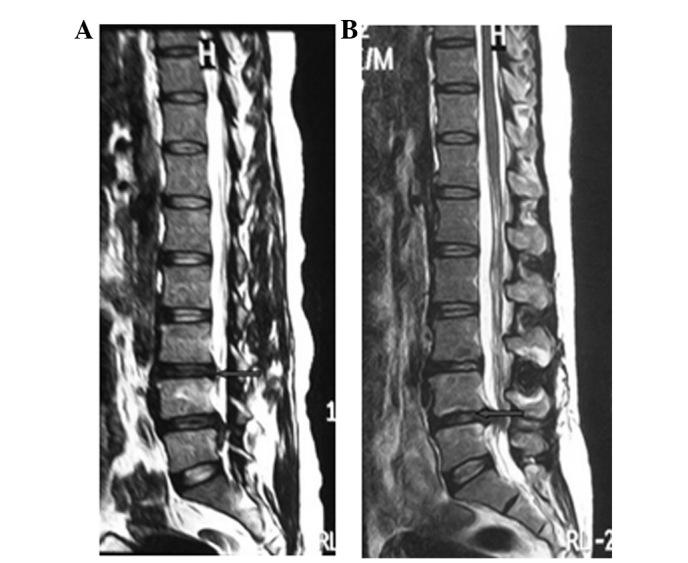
(A) Pre-operative MRI and (B) post-operative MRI at 6 months showed signal changes of hydration in L3/4. (A) Since a pre-operative herniated disc was indicated in L3/4 and L4/5, L4/5 underwent excision of the intervertebral disc and L3/4 underwent in-space implantation. T2 images showed that the hydration signals of L3/4 and L4/5 were poorer than those of other intercalated discs. (B) Post-operative rechecks at 6 months suggested significant improvements in the hydration signals of L3/4. Although L4/5 suffered a herniated disk, it was considered a post-operative recurrence.
